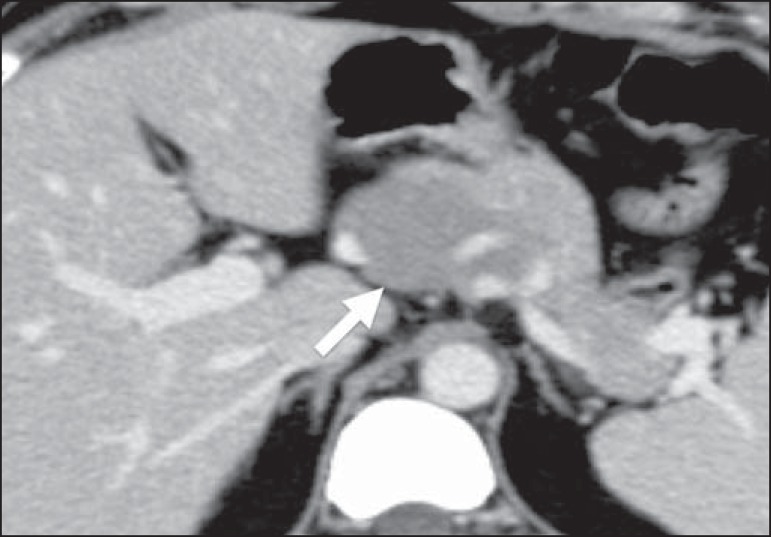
Pancreatic lymphoma. Contrast-enhanced axial CT of the abdomen, showing a
